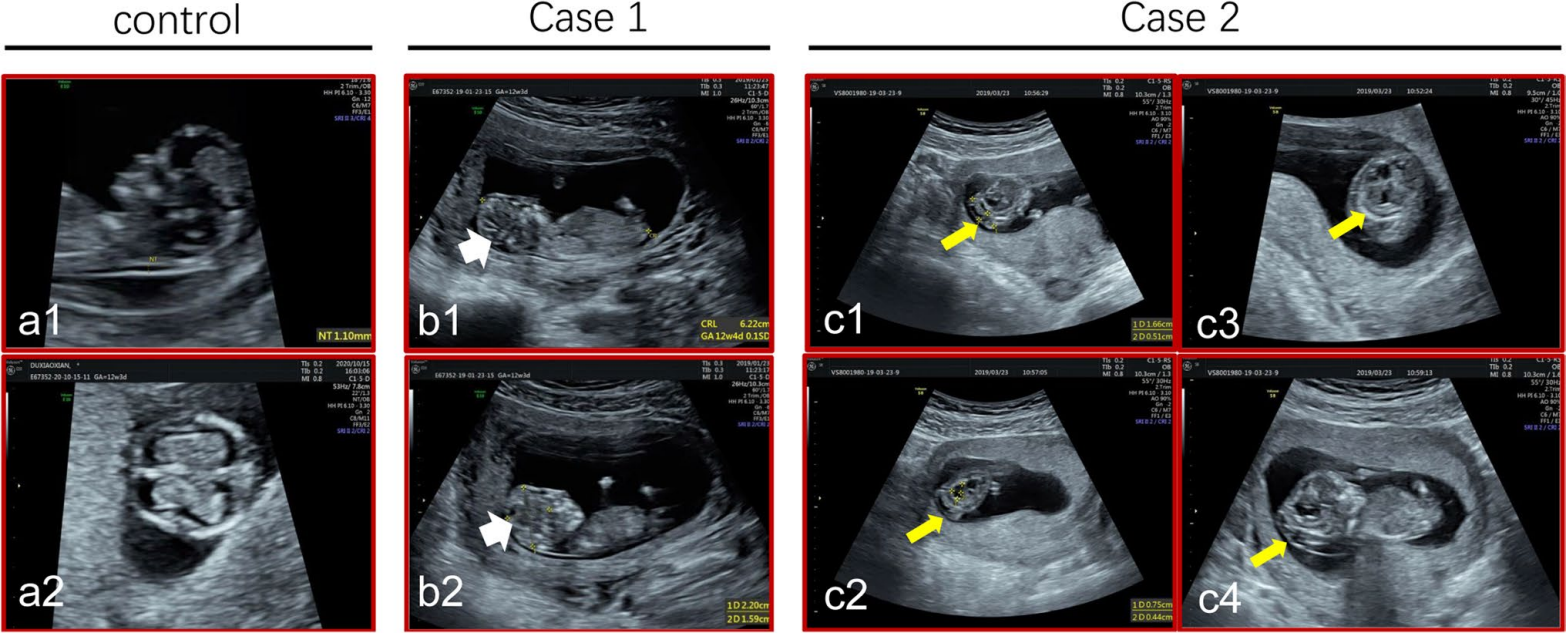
Prenatal ultrasound images. (a) Ultrasonography showed the brain of a normal fetus in sagittal section (a1) and in coronal section (a2). The fetus is of 12 weeks gestation. (b) Ultrasonography at 12th + 3 week of gestation of the brain of the fetal proband in case 1, in sagittal section. An apical hypoechoic bulge about 2.2 × 1.6 cm covered with entire envelope (occipital encephalocele?), incomplete skull, and mixed intracranial construction can be observed (white arrow in panel b1, b2). (c) Ultrasonography at 12th week of gestation of the fetal proband in case 2. An occipital mixed hypoechoic bulge about 1.4 × 0.7 cm in size (occipital encephalocele?) along with incomplete skull can be seen (yellow arrow in panel c1). The midline has disappeared and replaced by multiple anechoic area, one of which is about 0.8 × 0.4 cm
